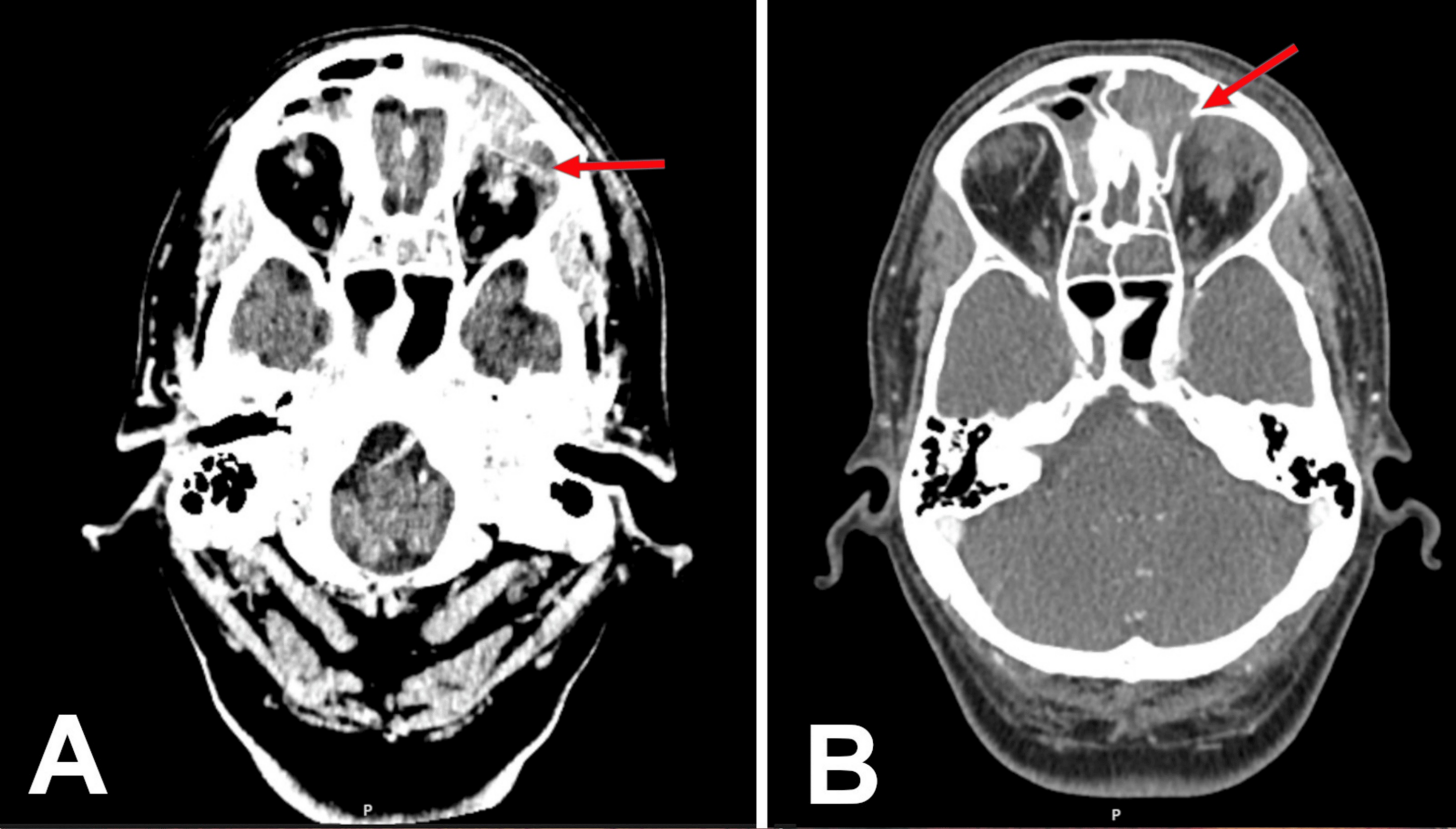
Axial CT imaging with red arrows demonstrating the extent of the pathology A: Contrast-enhanced CT brain. Demonstrating erosion of the frontal sinus wall with inflammatory material extending into the orbit (red arrow). B: Non-contrast-enhanced CT brain demonstrating erosion of the frontal sinus wall (red arrow) with inflammatory material extending into the orbit. The collection correlates with the clinically fluctuant superolateral eyelid swelling.
